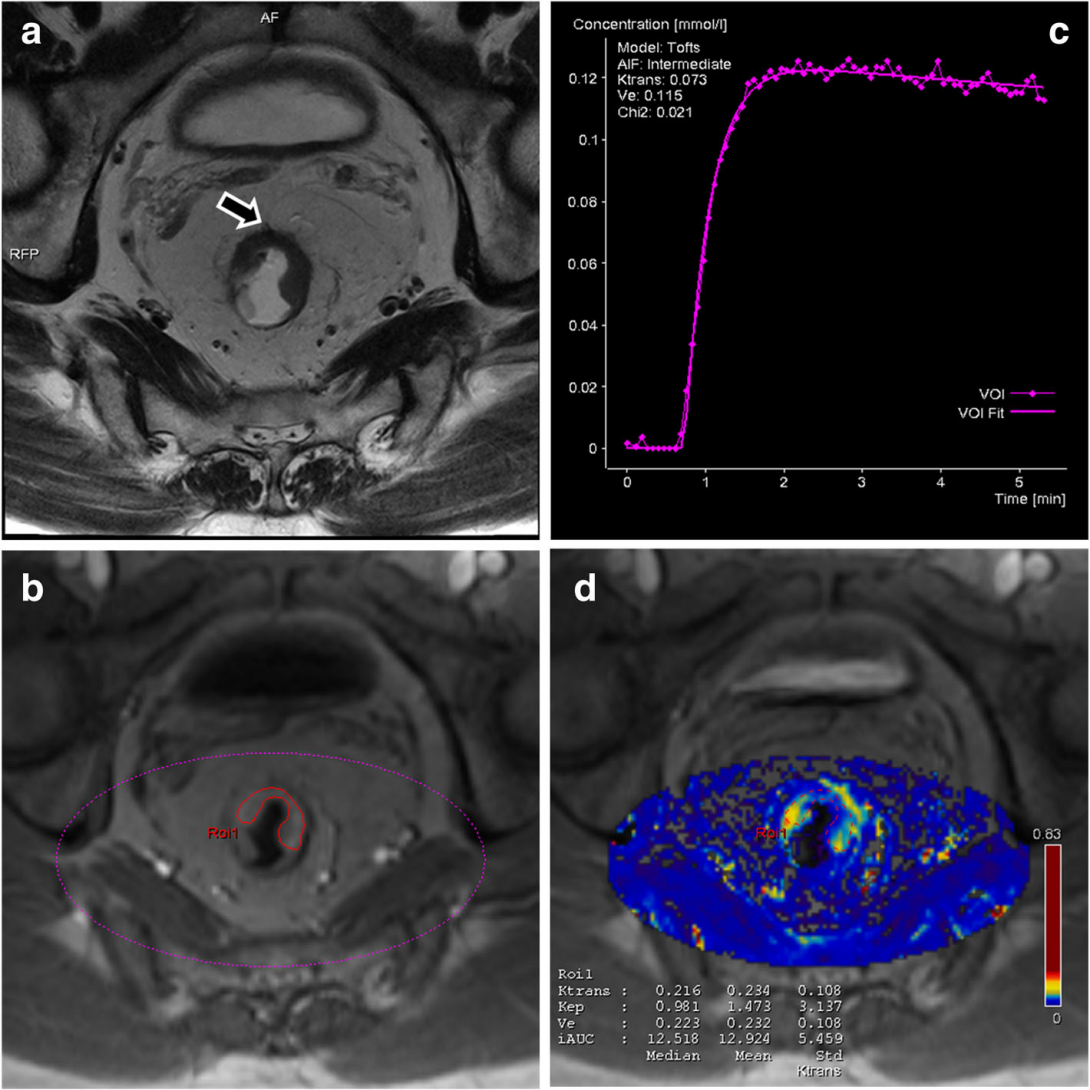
A patient with rectal adenocarcinoma (pT3N1, moderately differentiated). a Oblique axial high-resolution T2WI depicted the extramural vessels were in the vicinity of stranding rectal tumour, but these vessels had normal calibre, and there is no definite tumour signal within the vessels (arrow), indicating mrEMVI-negative status. b A VOI (pink dashed line) was selected on DCE-MRI, and (c) the AIF with the smallest chi-square value was than selected. b The ROI (red continuous line) was drawn along the edge of the tumour guided by corresponding (a) oblique axial high-resolution T2WI. d The ROI was copied to *K*^*trans*^ map, and the mean *K*^*trans*^ (0.234 min^− 1^), *k*_*ep*_ (1.473 min^− 1^) and *v*_*e*_ (0.232) values were obtained
